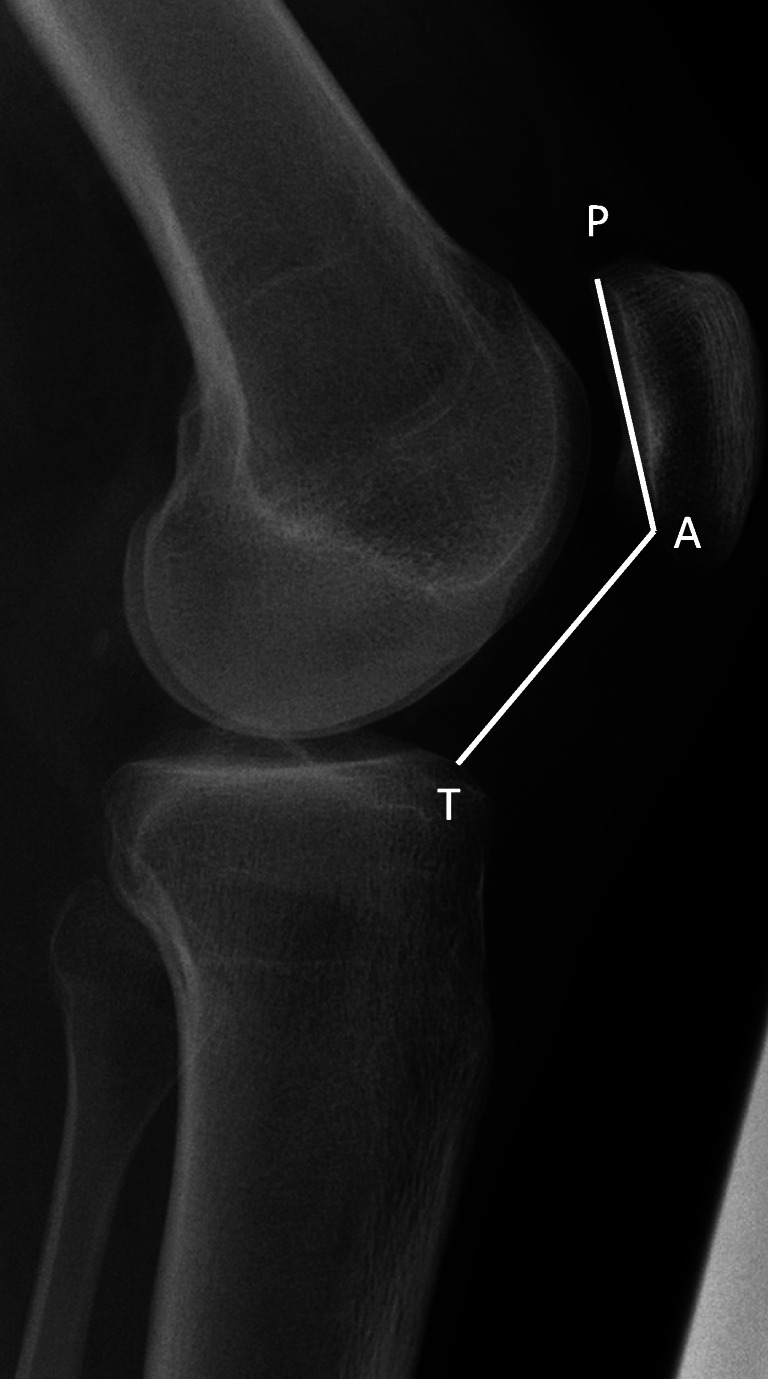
Calculation of the Caton-Dechamps Index on lateral radiographs with the knee in 20–30° flexion showing patella alta: the ratio of distance P to A and distance A to T is > 1.2; P and A, superior and inferior margins of the patellar articular surface; T, anterior tibial plateau
